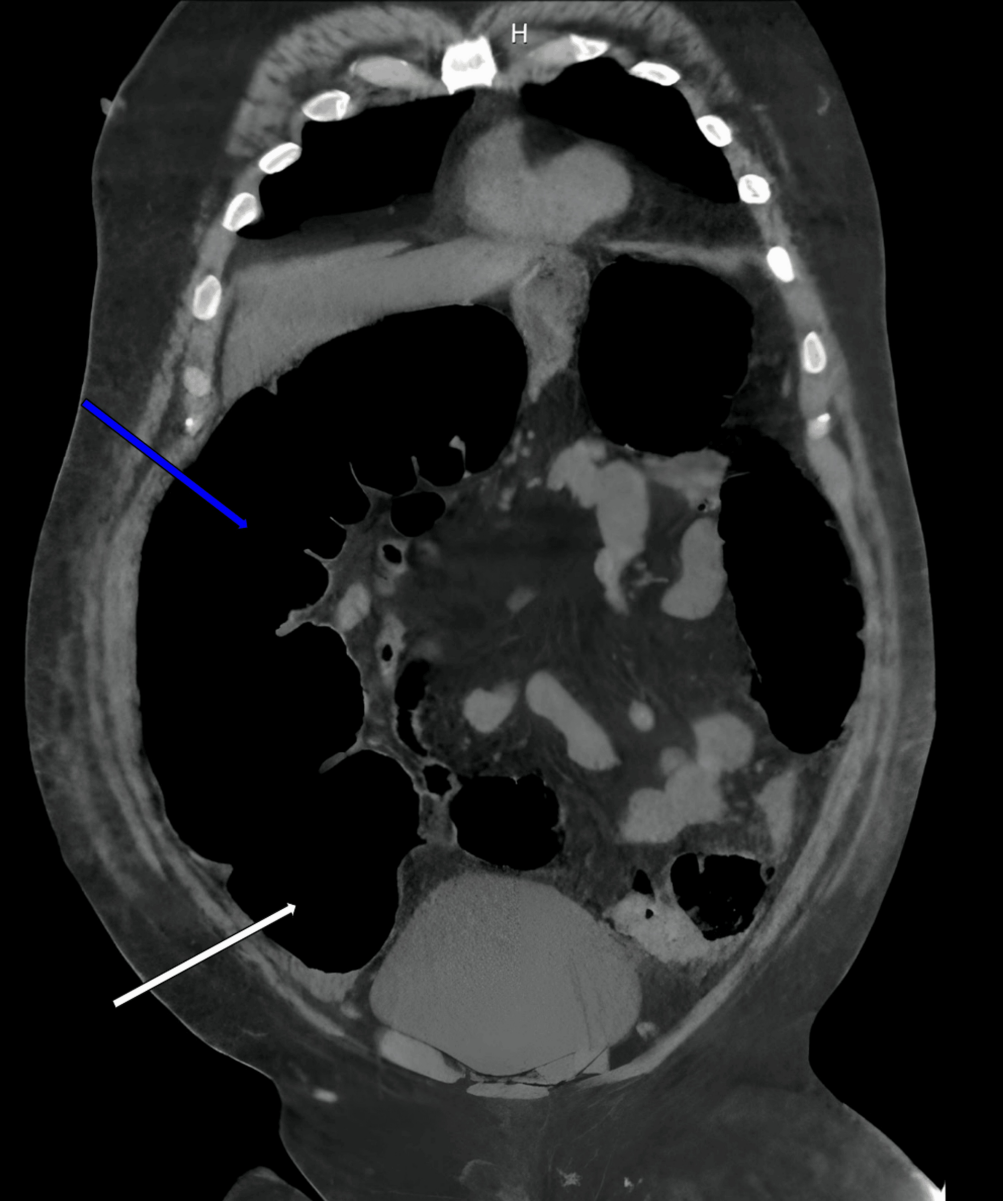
Coronal CT scan before colectomy showing dilated cecum (white arrow) and ascending colon (blue arrow) CT: computed tomography
